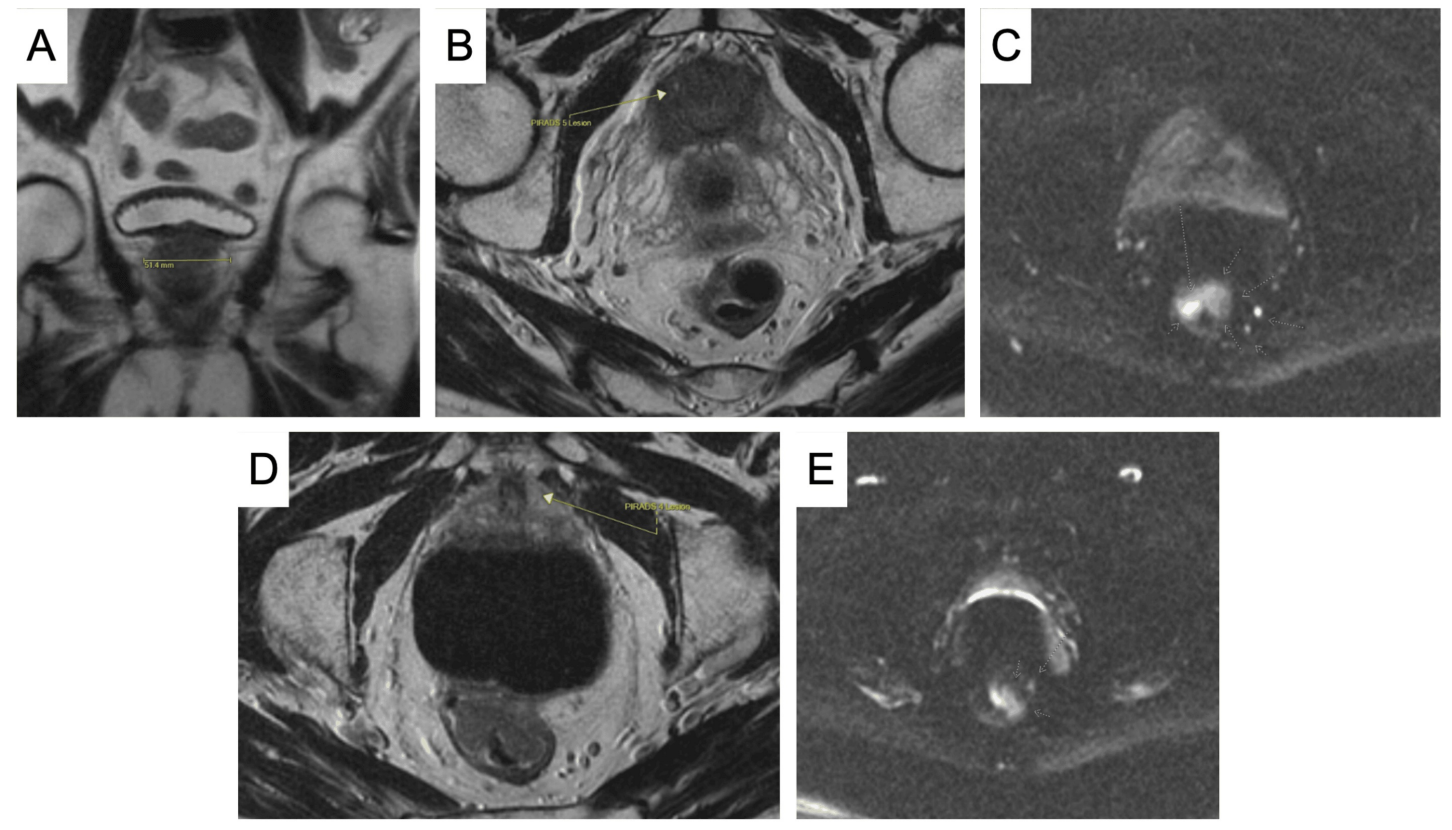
(A) Coronal MRI of the pelvis demonstrating a prostate measuring 5 cm transversely. (B) Axial MRI view and (C) diffusion-weighted image of a T2-weighted hypointense 1.7 cm nodule in the right mid-gland graded as PI-RADS 5. (D) Axial MRI view and (E) diffusion-weighted image of a T2-weighted hypointense 1 cm nodule arising from the midland to the apex of the left peripheral zone graded as PI-RADS 4. MRI, magnetic resonance imaging; PI-RADS, Prostate Imaging-Reporting and Data System
